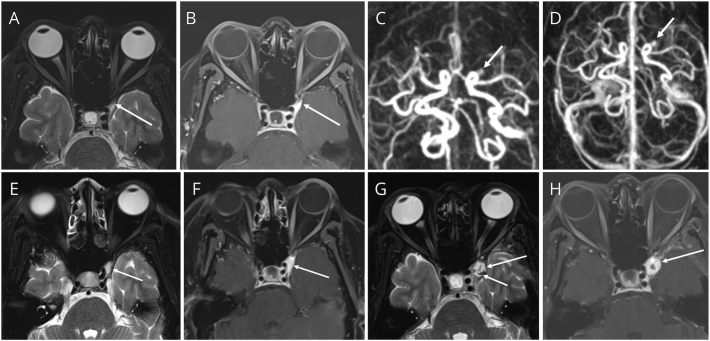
MRI orbits at initial presentation: axial image T2WI (a) TIW post contrast (b) showeing small heterogenous signal on T2WI and intensely enhancing lesion in the anterior left anterolateral cavernous sinus (arrows in a, and b), and dynamic post contrast MR angiography showing opacification in the late arteriographic phase (c), extending into the venous phase (d) (arrows in c, and d). MRI orbits axial image T2WI (e) TIW post contrast (f) showing progression in size (arrows in e, and f). Follow-up MRI follow up after 6 months: T2WI (g) showing further increase in the size, T2 heterogenous bright signal, with intralesional hypointensities (long and short arrows in in g respectively) in keeping with a hemorrhagic component and intense marginal enhancement on T1WI post contrast image (arrow in h).
